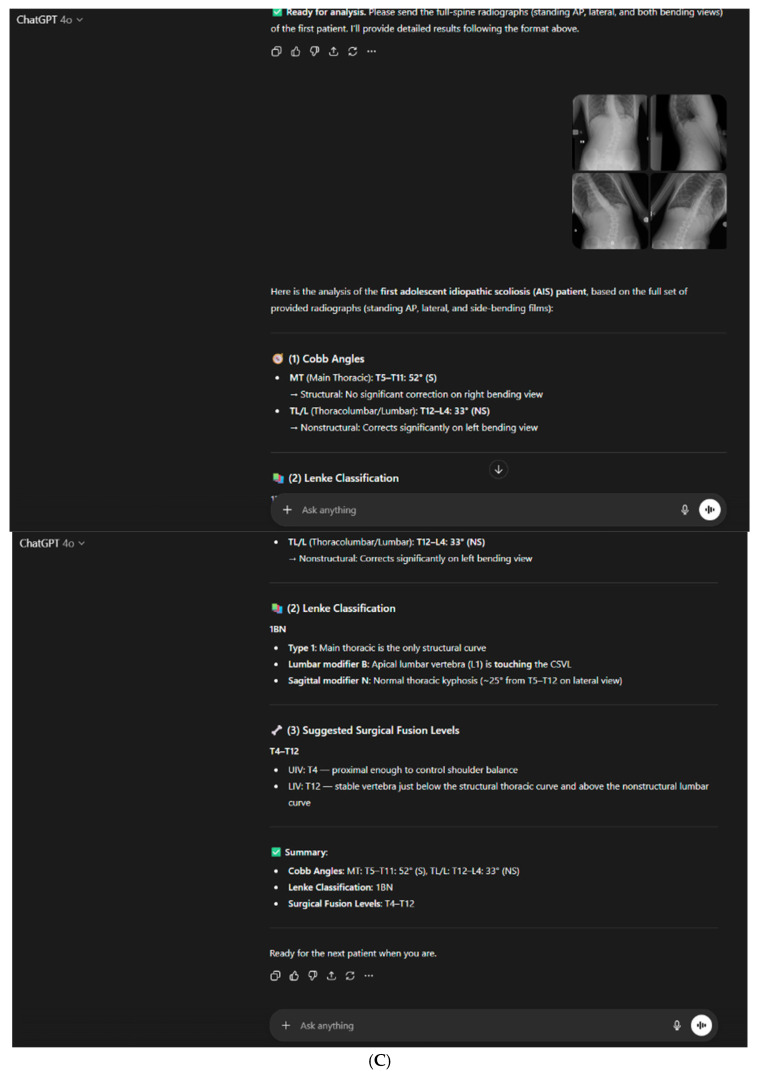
Example of a confident failure (“hallucination”) by the multimodal LLM: (A) Standing posteroanterior radiograph of an adolescent idiopathic scoliosis case unanimously classified as Lenke type 5 by two orthopedic surgeons. (B) Prompt provided to the multimodal LLM requesting Lenke classification. (C) Model-generated response confidently classifying the curve as Lenke type 1, accompanied by anatomically inconsistent reasoning.
